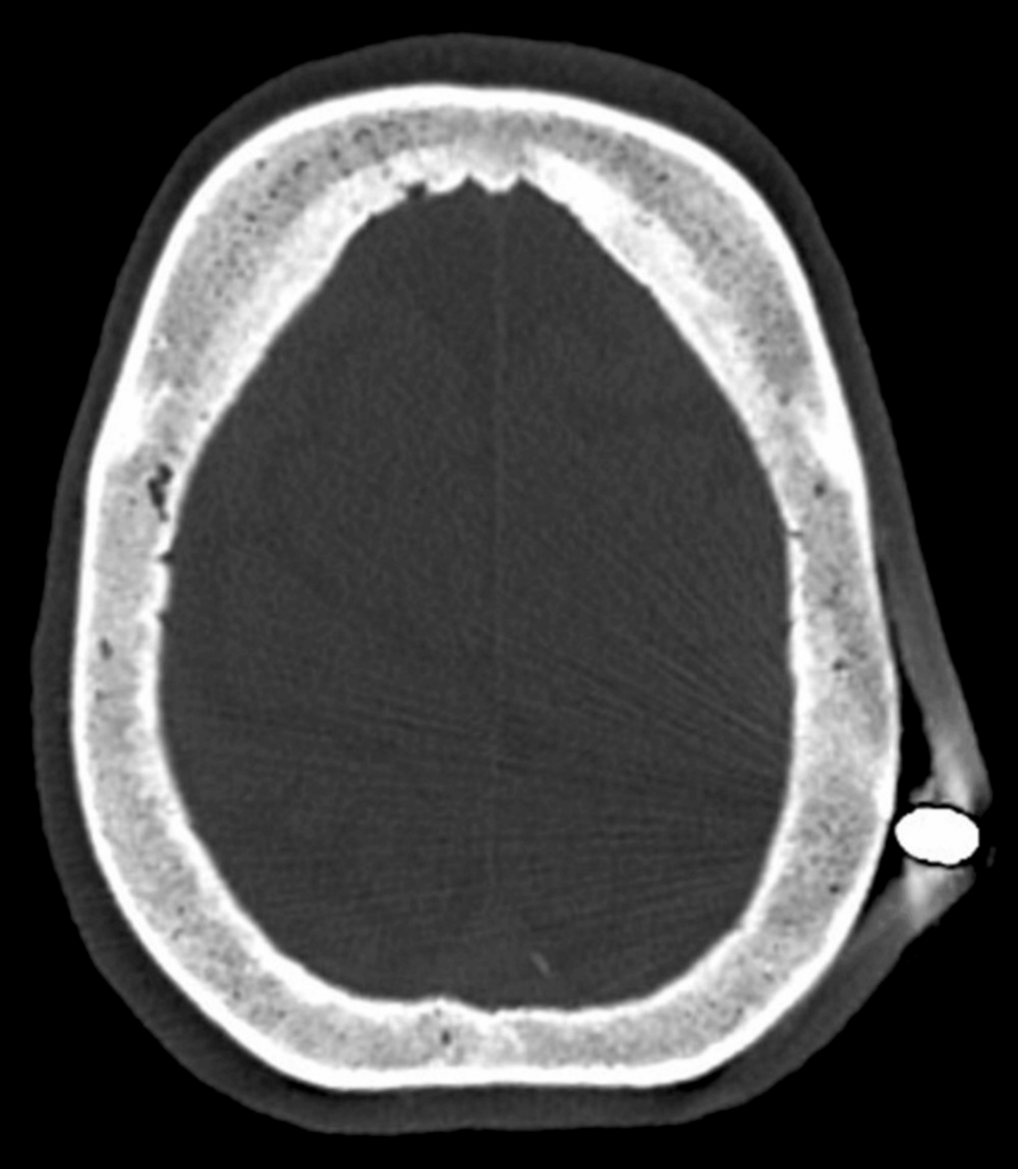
Axial head CT. Soft tissue injury over the left parietal region with the arrow tip in contact with the external cortical bone, without evidence of intracranial involvement. CT: computed tomography
